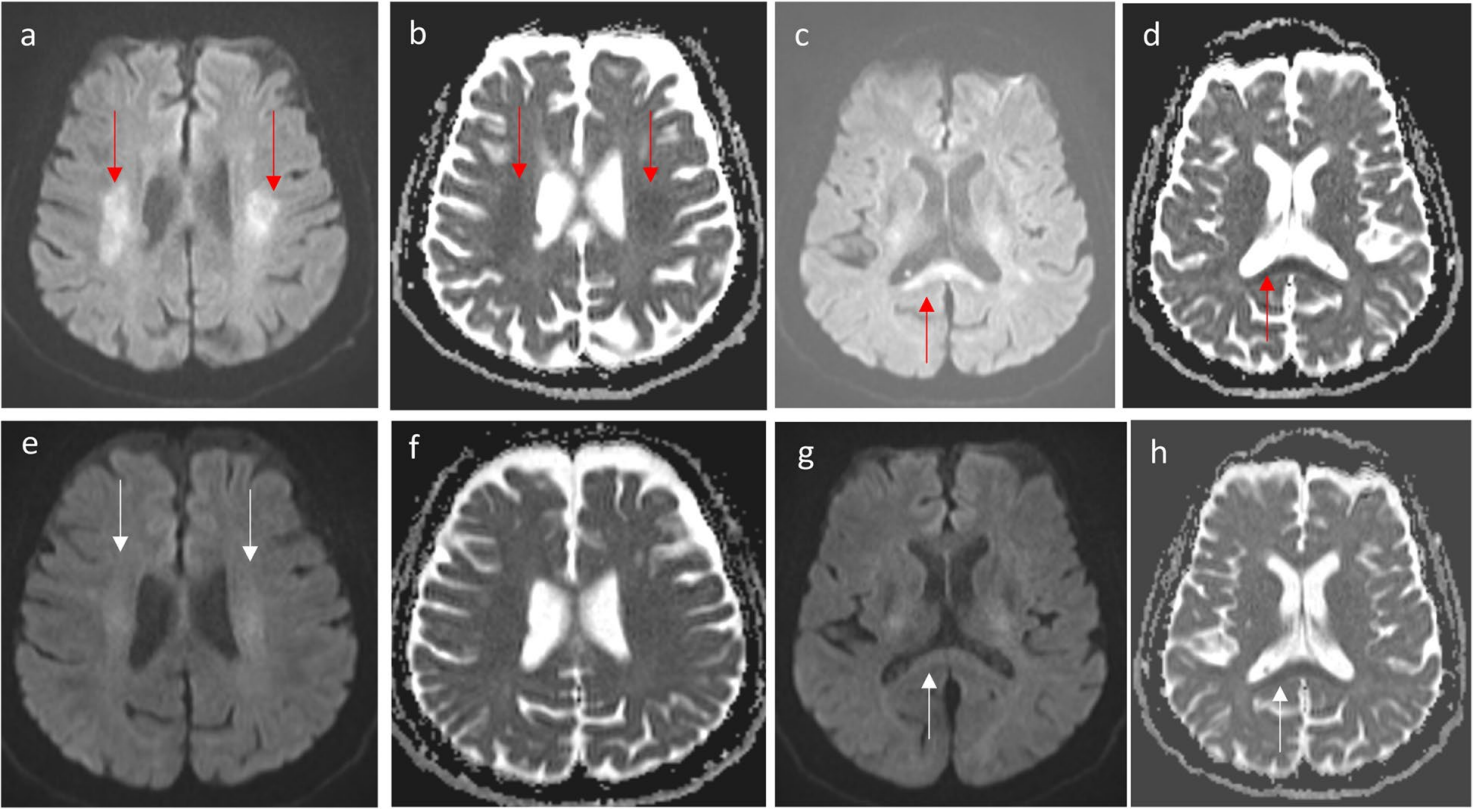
Capecitabine-induced leukoencephalopathy*.* An elderly patient on capecitabine for colon adenocarcinoma presented with transient expressive dysphasia and unsteady gait. Axial diffusion-weighted imaging (DWI) (a, c) and axial apparent diffusion coefficient (ADC) (b, d) MRI demonstrates restricted diffusion in the corona radiata (arrows in a, b) and splenium of the corpus callosum (arrows in c and d). These findings are compatible with capecitabine-induced leukoencephalopathy. A follow-up MRI after cessation of capecitabine administration showed near-complete resolution of the diffusion changes in bilateral corona radiata (arrows in e) and splenium of the corpus callosum (arrows in g and h) on the axial DWI (e, g) and ADC (f, h) images
